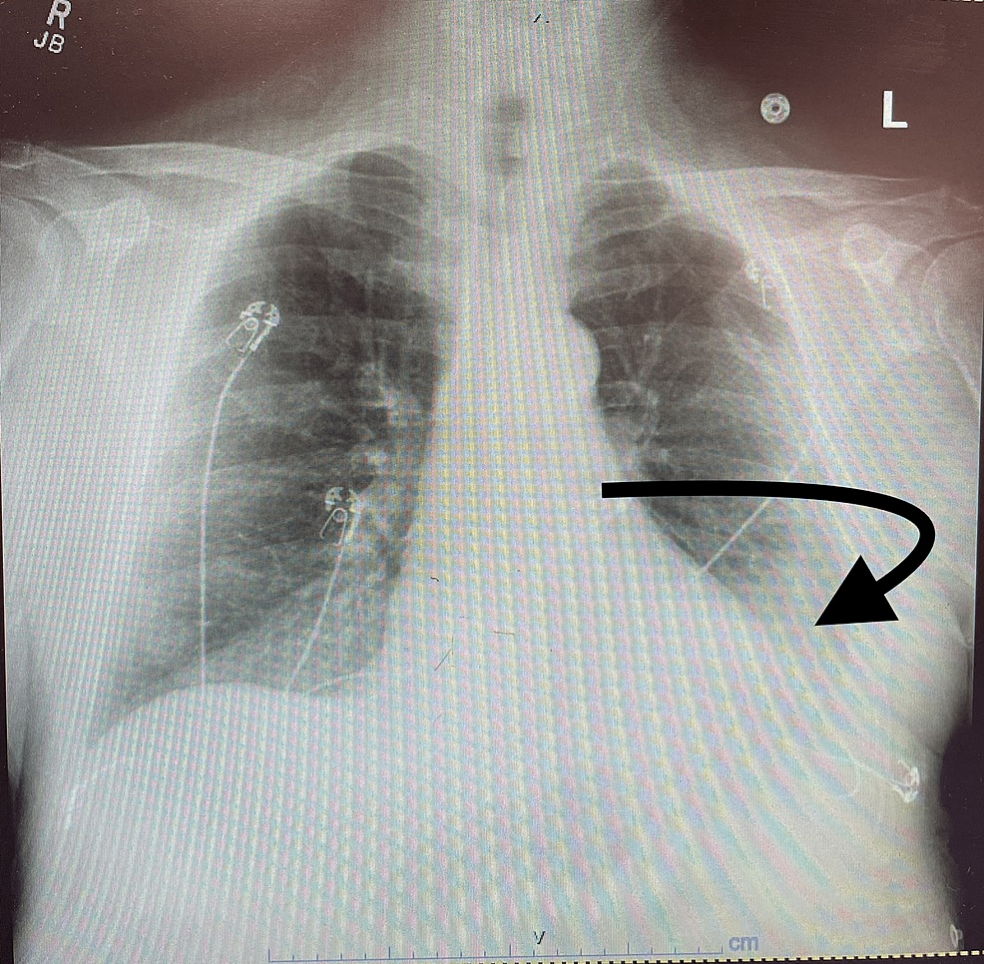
Chest x-ray showing mild to moderate left pleural effusion, and atelectasis in the left lung base.
